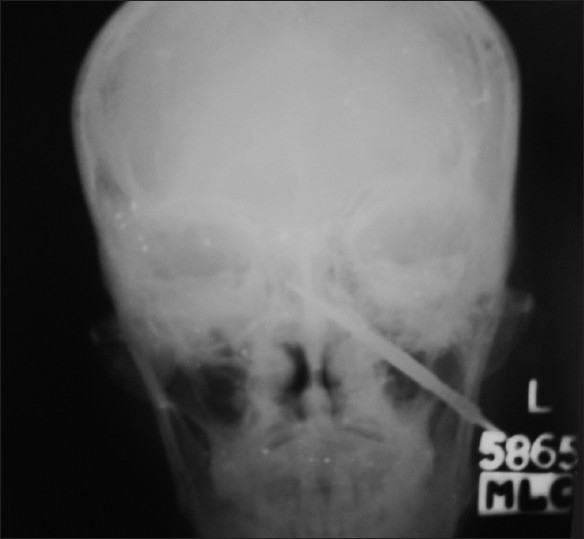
X-ray of the skull showing a 10-cm-long arrowhead penetrating the base of the skull from below the mastoid process obliquely and reaching inside the middle cranial fossa
